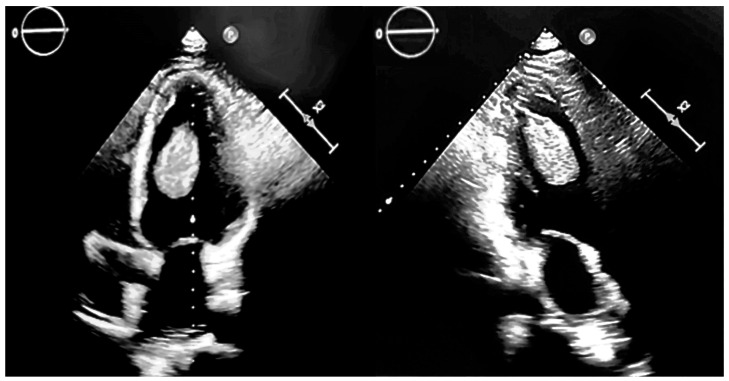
Echocardiographic appearance of a pediculated apical left ventricular tumor.
